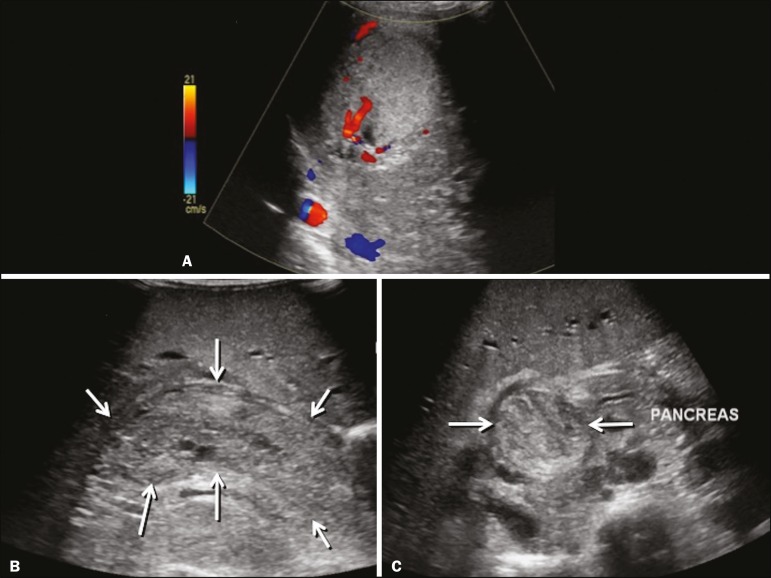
Biliary rhabdomyosarcoma in a 4-year-old girl with a three-week history of jaundice, acholic stools, abdominal pain, and pruritus, who was referred for investigation of a suspected cyst in the common bile duct. A: Doppler ultrasound of the abdomen, showing a hyperechoic solid liver lesion with central and peripheral vascularization. B,C: Lesion in the lumen of the common bile duct (longitudinal and transverse sections, respectively), occupying and significantly increasing its caliber (arrows).The lesion is solid and hyperechoic, containing cystic foci. The image in C also shows the obstructive effect produced by the lesion with repercussions for the pancreatic duct, the caliber of which is slightly increased. The mass was surgically resected, and the pathology study indicated biliary rhabdomyosarcoma with hepatic invasion.
